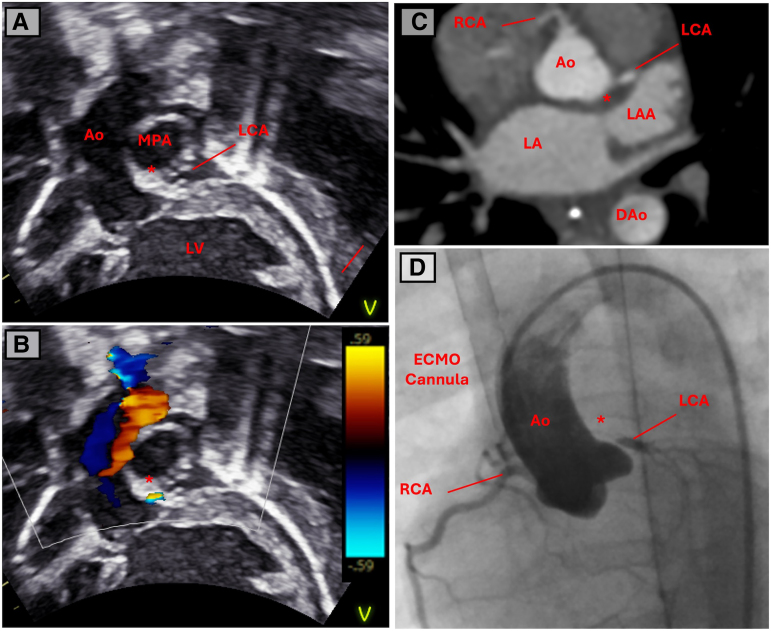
Imaging performed on a 2-month-old with dilated cardiomyopathy on venous-arterial extracorporeal membrane oxygenation support after an unsuccessful decannulation wean. *(A)* 2D subcostal left ventricular outflow view with the distal left coronary artery visible and *(B)* colour Doppler demonstrating turbulent flow between aortic root and visible left coronary artery (asterisk). *(C)* An axial computed tomography image of the aortic root at the level of the coronary artery origin. There is a suspicion of a focal occlusion to flow between the aortic root and left main coronary artery. (*D*) Cardiac angiogram demonstrating a clear stenotic portion of the origin of the left main coronary artery.
